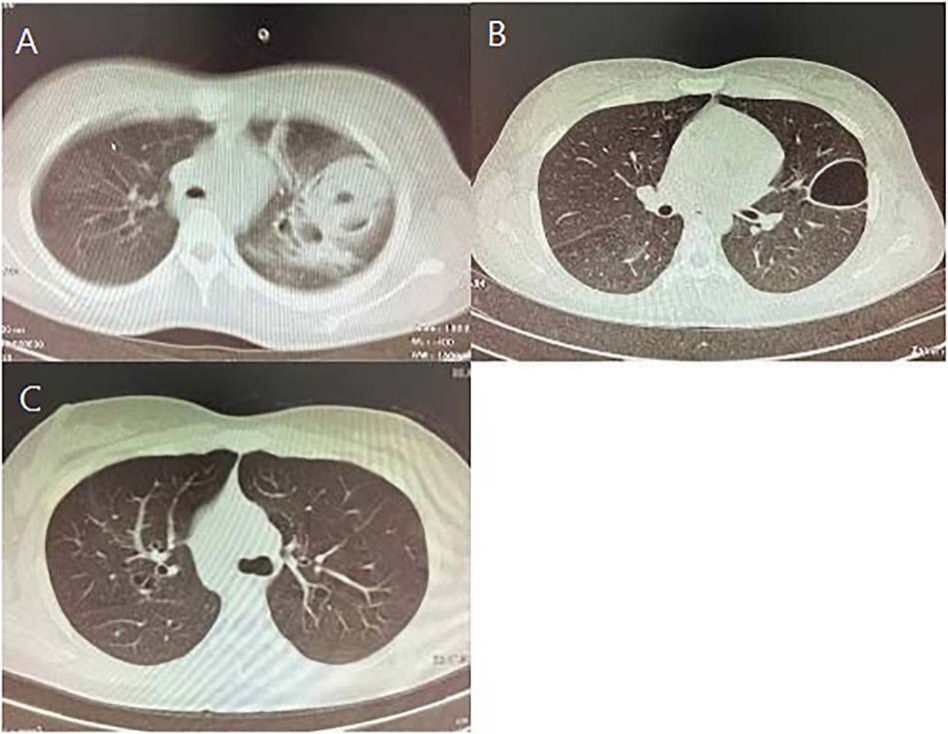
Computed tomography of the chest taken within treatment periods with Voriconazole. (A) Right after bronchoscopy (B) One month after hospitalization (C) 15 month after treatment.
